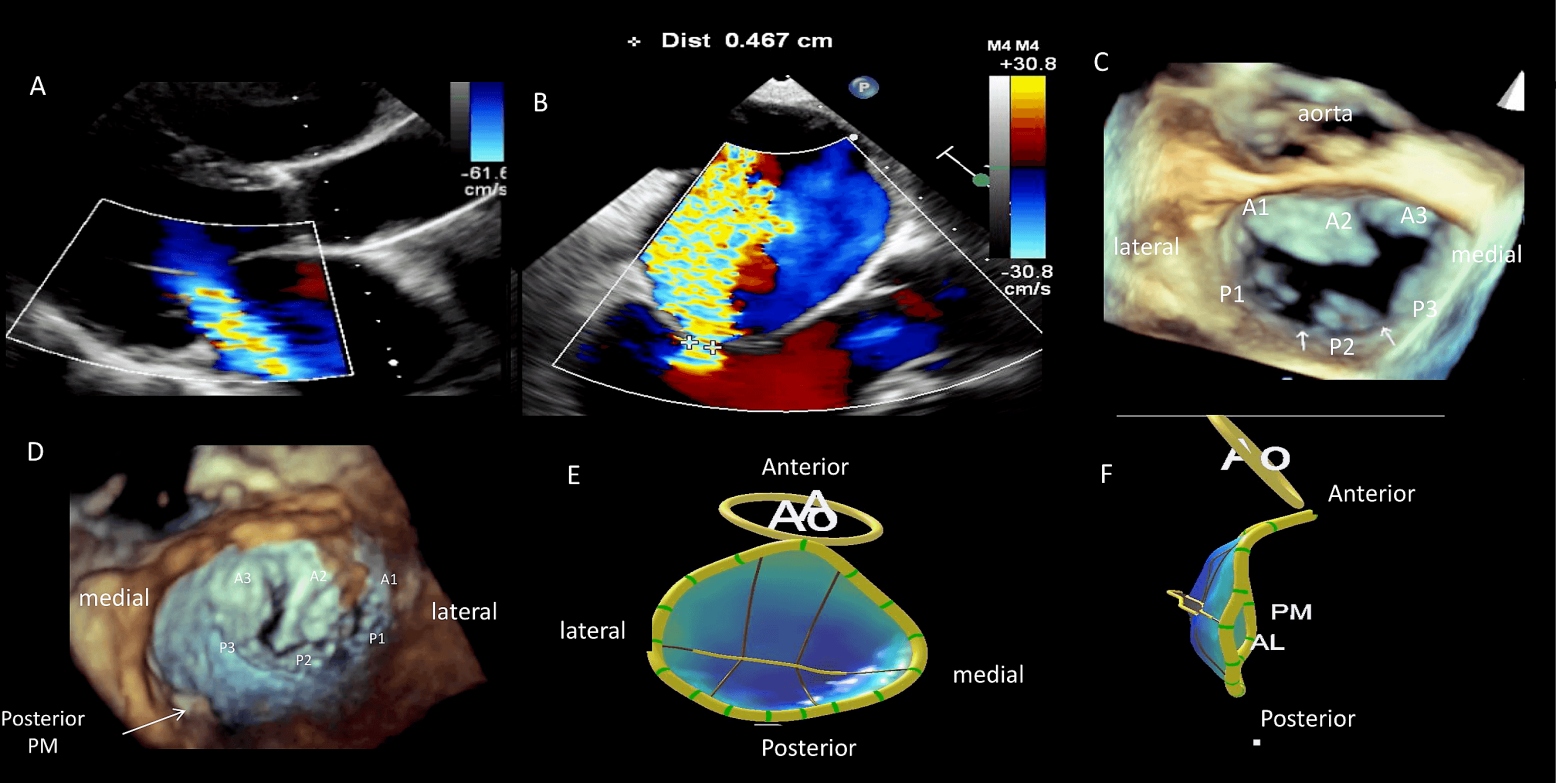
Multimodality echocardiographic imaging of secondary ischemic
